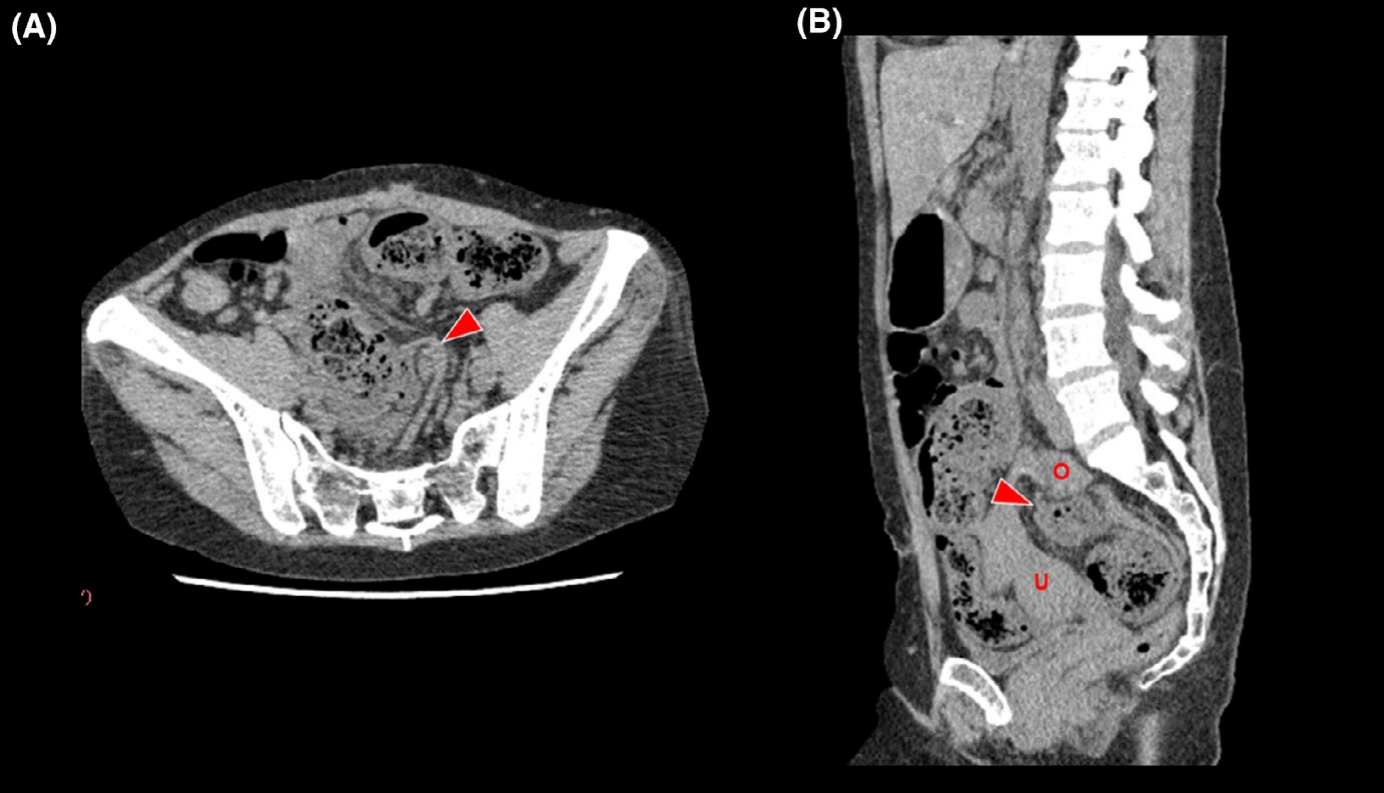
A, An axial view of a computed tomography (CT) scan showed stenosis of the sigmoid colon (arrowhead). B, A sagittal view of the CT scan showed the protrusion of the sigmoid colon (arrowhead) between the uterus (U) and ovary (O)
